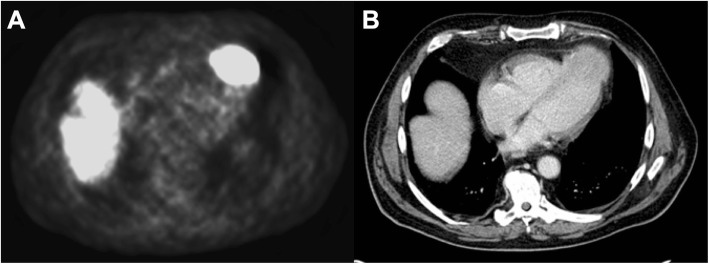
Patient example with a myocardial metastasis located at the apex evident on 68Ga-DOTATATE PET (a) and CT (b). A 77-year-old male patient with a G3 neuroendocrine tumor of unknown origin with a myocardial metastasis to the apex of the heart. Strong 68Ga-DOTATATE tracer uptake (a) correlates to the morphologic mass detected on CT imaging (b)
